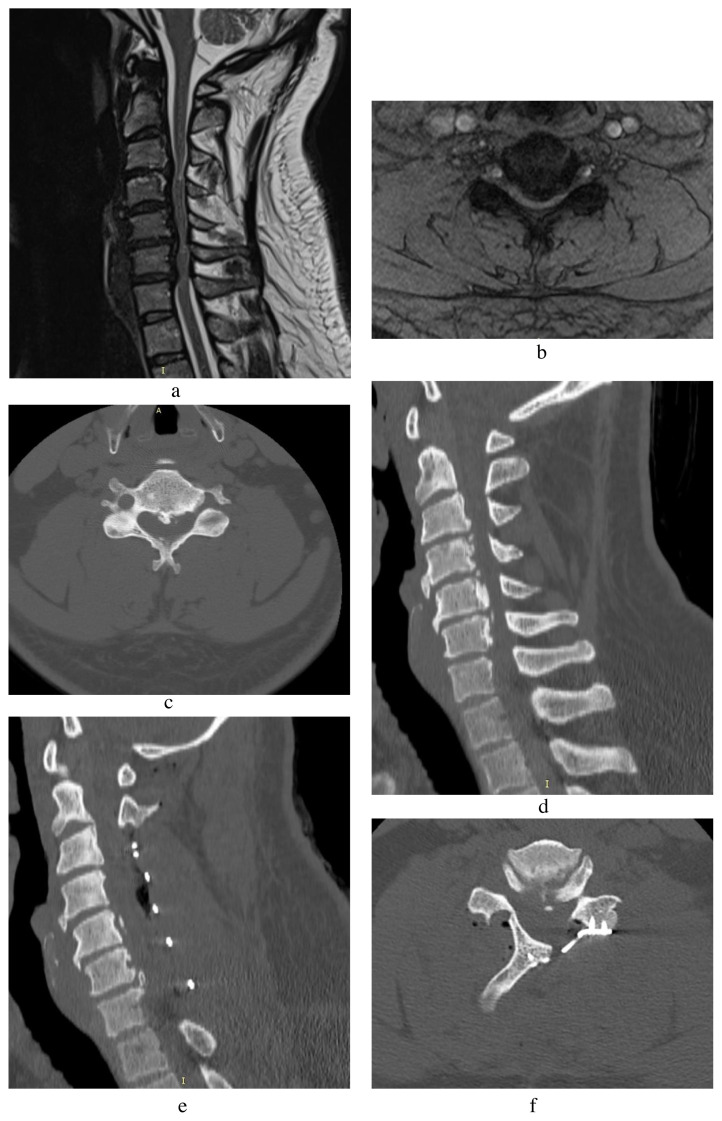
Sagittal T2-weighted MRI of a patient is demonstrating severe cervical spinal canal stenosis from C3 to C7, especially C3–4 and C5–6 due to degenerative disc protrusions and narrow spinal canal, causes complete CSF erasure and spinal cord compression at these levels. There is a high T2 signal noted within the cord due to both edema and myelomalacia. B. Axial T2-weighted MRI at C5–6 level is demonstrating severe spinal canal stenosis. C. Axial CT at C5–6 level is demonstrating intense calcification and spinal cord compression. D. Preoperative sagittal CT is demonstrating dense osteophyte formation which is apparent in both anterior and posteriorly. E. Postoperative sagittal CT is showing enlargement and space gained in the spinal canal. F. Postoperative axial CT is showing open door laminoplasty with titanium screws and plate and enlargement of the spinal canal.
